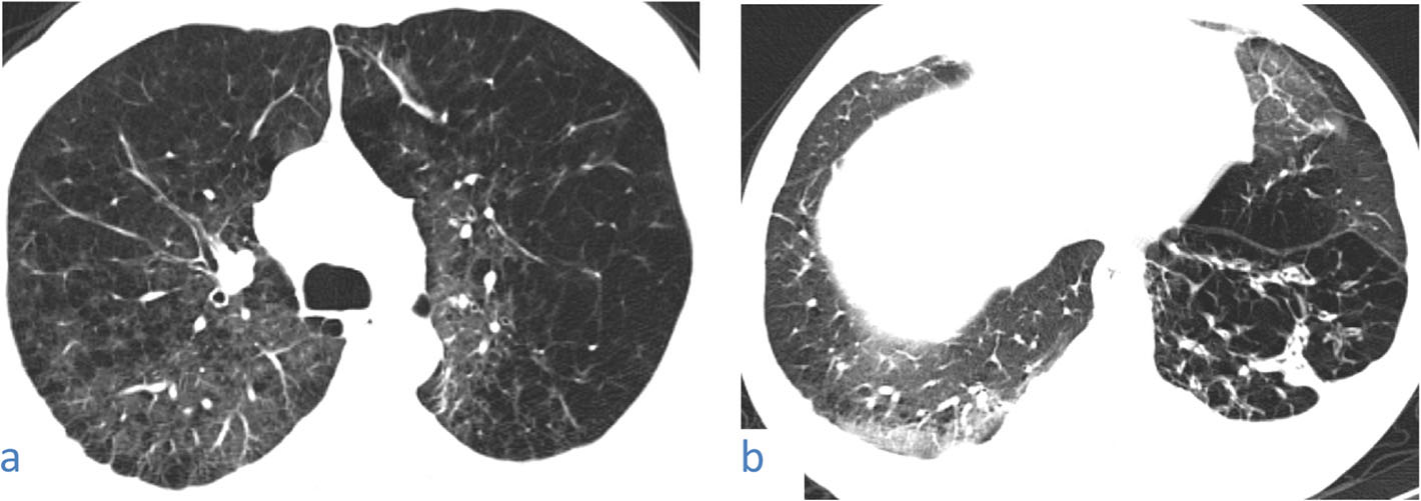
A 48-year-old male smoker with COVID-19. Axial CT image (a) shows marked upper lobes centrilobular emphysema with subpleural and patchy ground glass opacification (b) shows right lower lobe peripheral ground glass opacity. Vascular thickening is seen related to lesions. Left lower lobe shows architectural distortion with fibrous stripes
